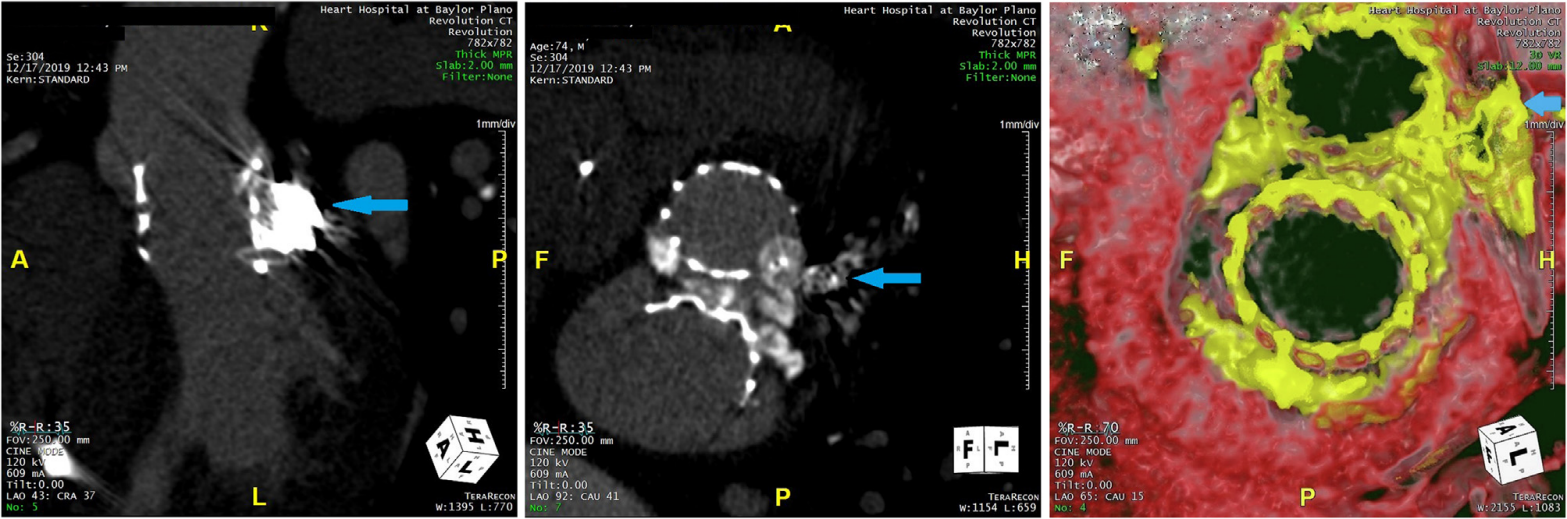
Follow-up CT heart 6 months after intervention showing Sapien valves with occlusion of pseudoaneurysm (arrows) by Amplatzer Plug and coils (left, middle). 3 dimensional CT reconstruction showing Sapien valves (aortic valve-top, mitral valve-bottom) with closure of pseudoaneurysm (arrow, right). Abbreviation: CT, computed tomography.
